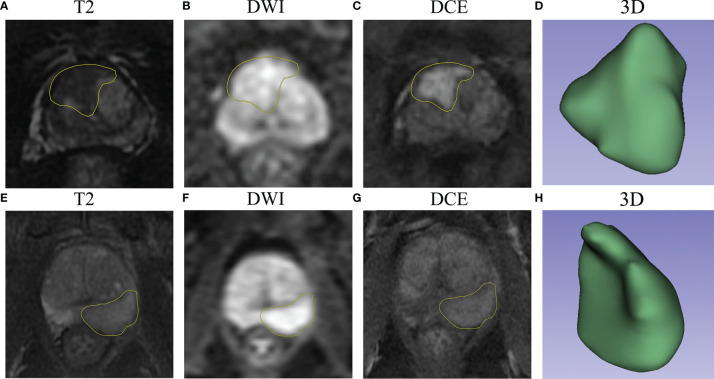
The examples of VOI delineation on MP-MRI. (A–D) A 66-year-old patient was pathologically diagnosed as PNI positive with a typical abnormal signal lesion in the right front of the prostate. (E–H) A 70-year-old patient was diagnosed as PNI negative with a lesion located in the left peripheral zone, and the DCE sequence showed moderate enhancement. (D, H) represent the 3-dimensional reconstruction of the VOI.
